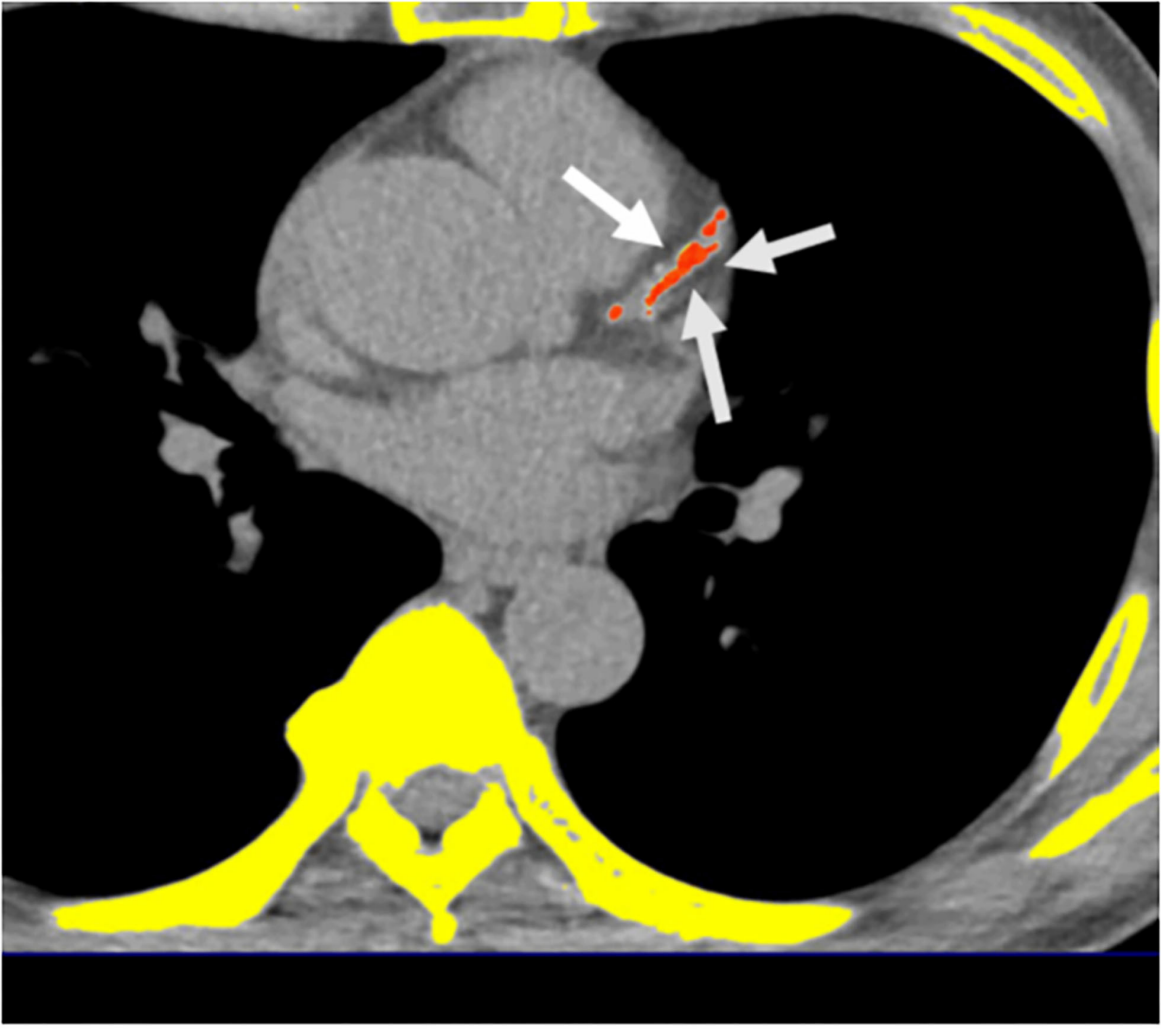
Multidetector computed tomography (MDCT) demonstrated the quantification of CACS. Semi-automatic quantification of CACS burden using Agatston scoring. *Orange color regions indicate visceral fat tissue. White arrows indicate coronary calcification lesions.
